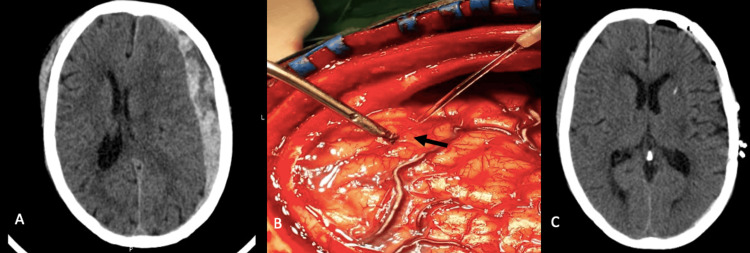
(A) Head CT scan with ASDH and midline shift. (B) Clipped cortical microaneurysm (arrow). (C) Postoperative head CT scan. CT, computed tomography; ASDH, acute subdural hematoma
